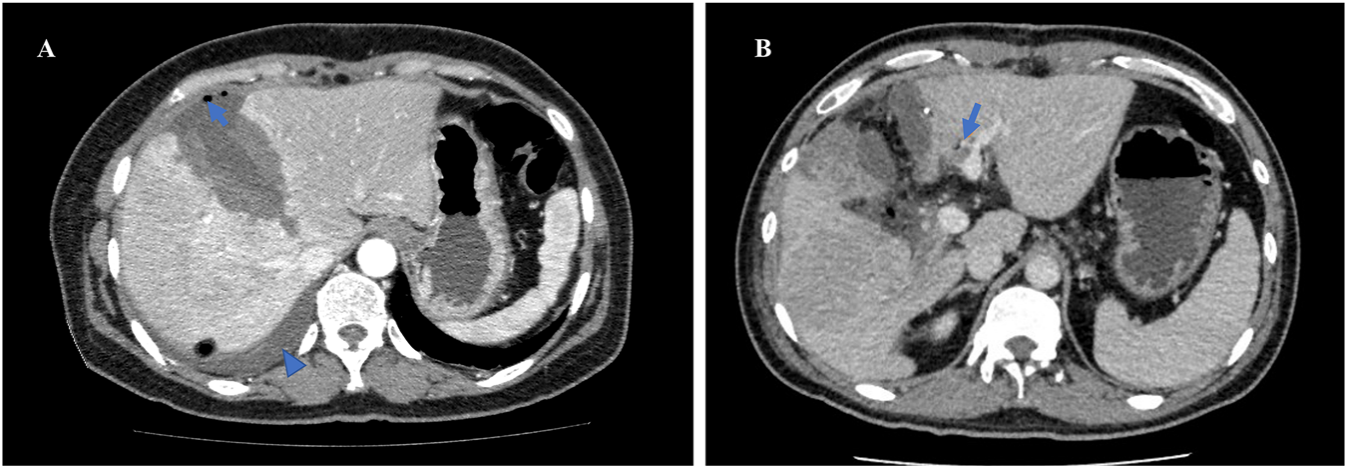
Normal findings and complications after stage 1. A A 57-year-old female patient, axial enhanced CT after ALPPS Stage 1 showed normal postoperative findings, including heterogeneous hyperenhancement of the liver parenchyma in the right lobe and at the cut edge, as well as a small amount of gas (arrow) and liquid (arrowhead) in the surgical area and right pleural cavity. B A 38-year-old male patient, axial enhanced CT after ALPPS Stage 1 in another patient, showed abnormal thrombosis in the left internal portal vein branch (arrow)
